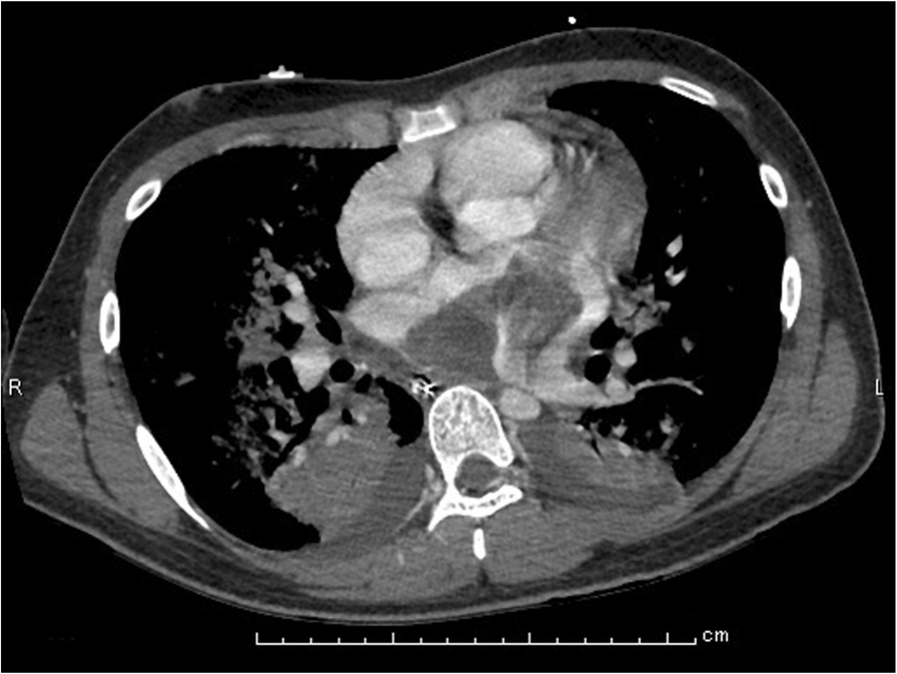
Pre-operative CT image. An enhanced CT scan showed lung congestion and intra-atrial tumor occupying the entire left atrial cavity
